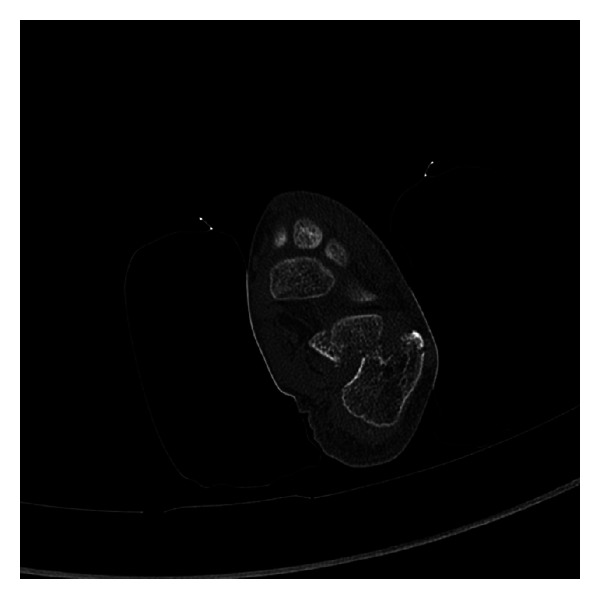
CT scan revealing fracture through body of calcaneus.
